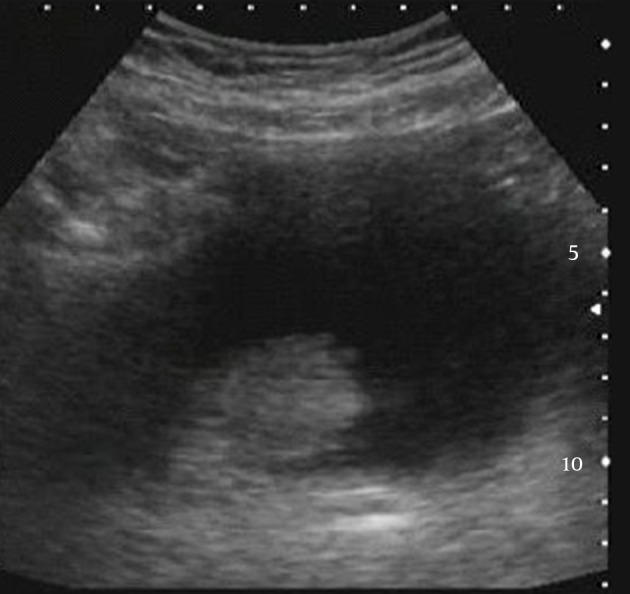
Transabdominal Ultrasound: Abnormal Thickening of Luminal Bladder, With Presence of Nodules.
